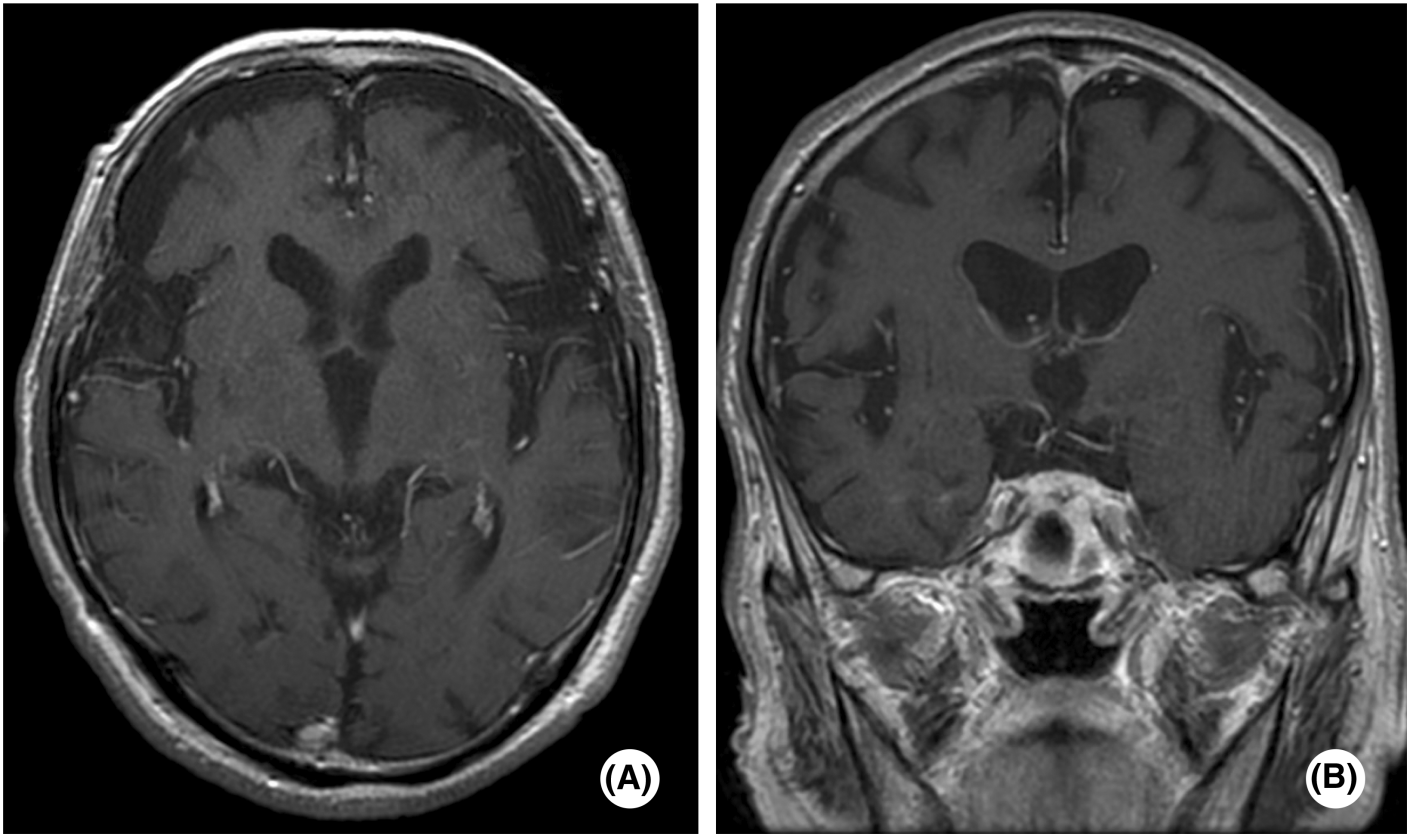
Contrast‐enhanced magnetic resonance imaging (MRI) of head computed tomography (CT).
